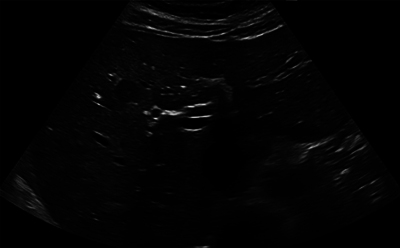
Ultrasound of the liver showing hypoechogenic nodular lesions.
